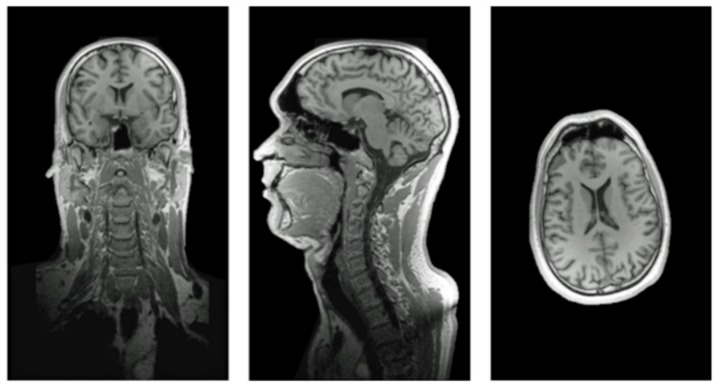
In vivo MR images. First in vivo measurements (MPRAGE images) using the complete device (i.e., RF coil integrated with TxS system).
